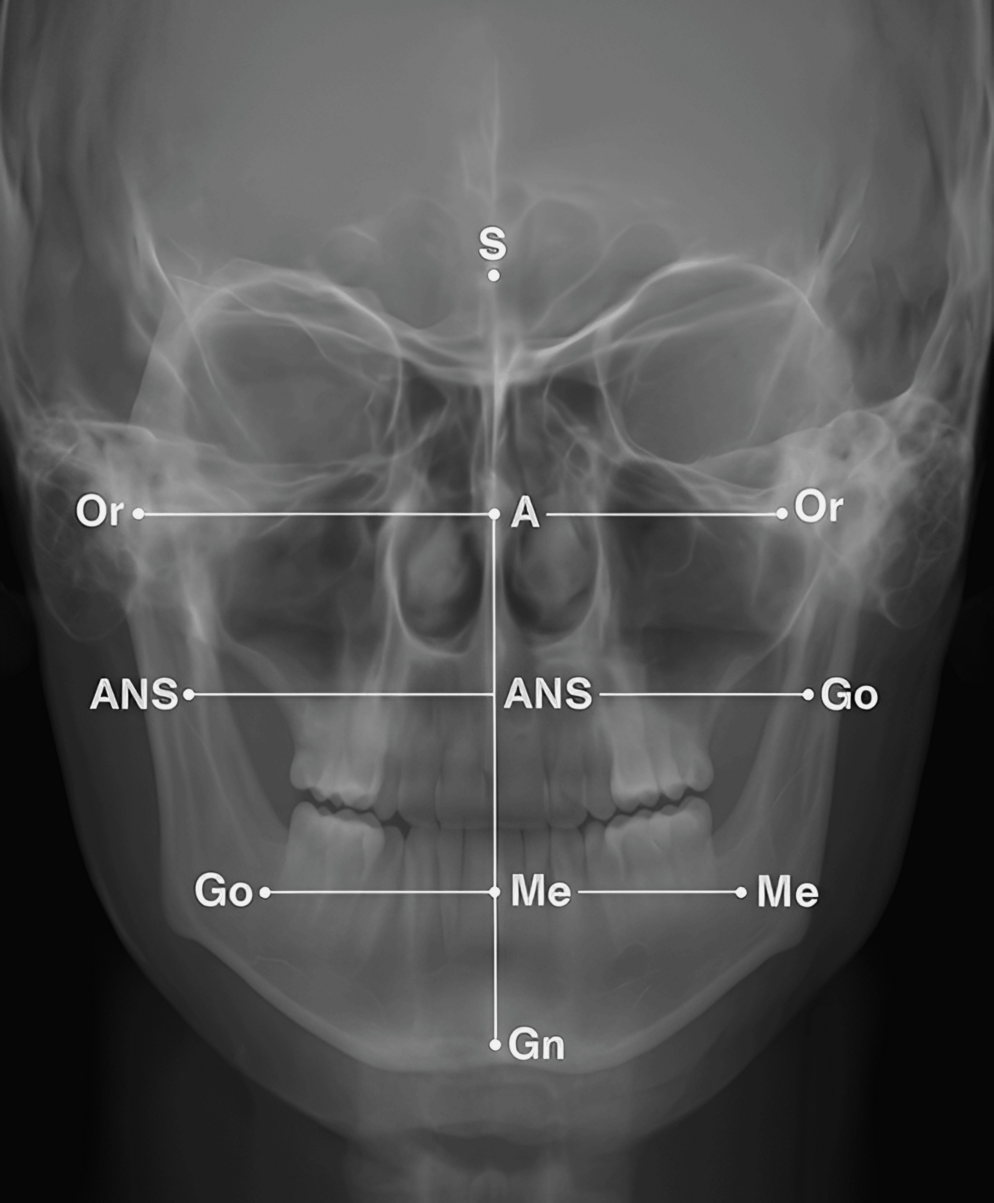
Frontal cephalometric radiograph showing key anatomical landmarks used in morphometric analysis. S: Sella; N: Nasion; Or: Orbitale; A: Point A (subspinale); ANS: Anterior Nasal Spine; Go: Gonion; Me: Menton; Gn: Gnathion.
